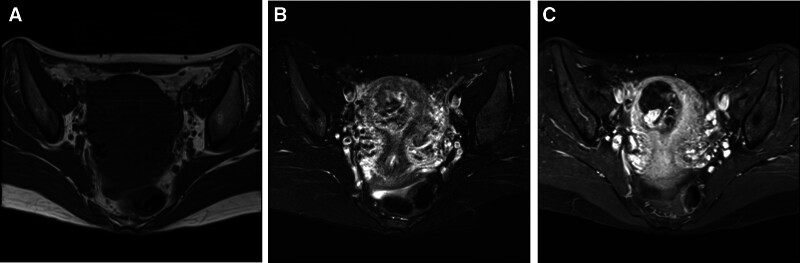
Magnetic resonance imaging (MRI) showing uterine arteriovenous fistula (UAVF). Axial T1-weighted MRI (A) shows a lesion in the right uterine angle and mixed isohyperintensity with a few high signals in it. Axial T2-weighted MRI (B) shows the lesion has mixed hyperintensity and “honeycomb sign” can be seen. Axial T1-weighted enhanced MRI (C) shows that the lesion is cystic. The cystic component is not enhanced, and the solid component is significant strengthening.
